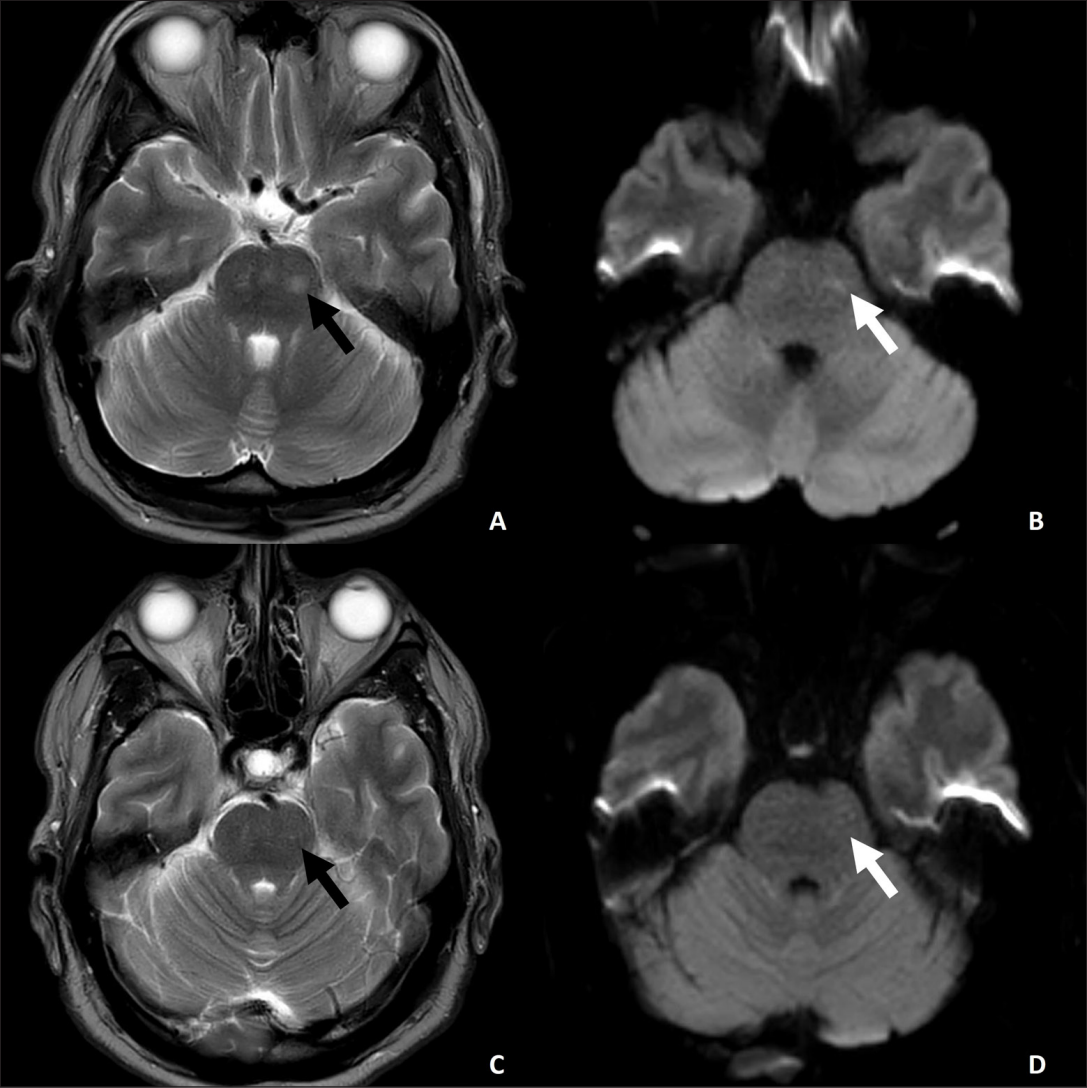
Magnetic resonance imaging of the pons. (A) Axial T2-weighted image shows bilateral, ill-defined hyperintensities in the central pons. (B) Diffusion-weighted imaging (b1000) demonstrates no evidence of restricted diffusion, and the corresponding ADC map (not shown) indicates preserved diffusion, suggesting a vasogenic component. (C) and (D) Follow-up imaging four weeks later shows complete resolution of the hyperintensities. The imaging pattern, in combination with clinical reversibility, is consistent with reversible pontine dysfunction due to osmotic injury. While the findings favor vasogenic edema, a mild form of osmotic demyelination syndrome (ODS) cannot be entirely excluded.
